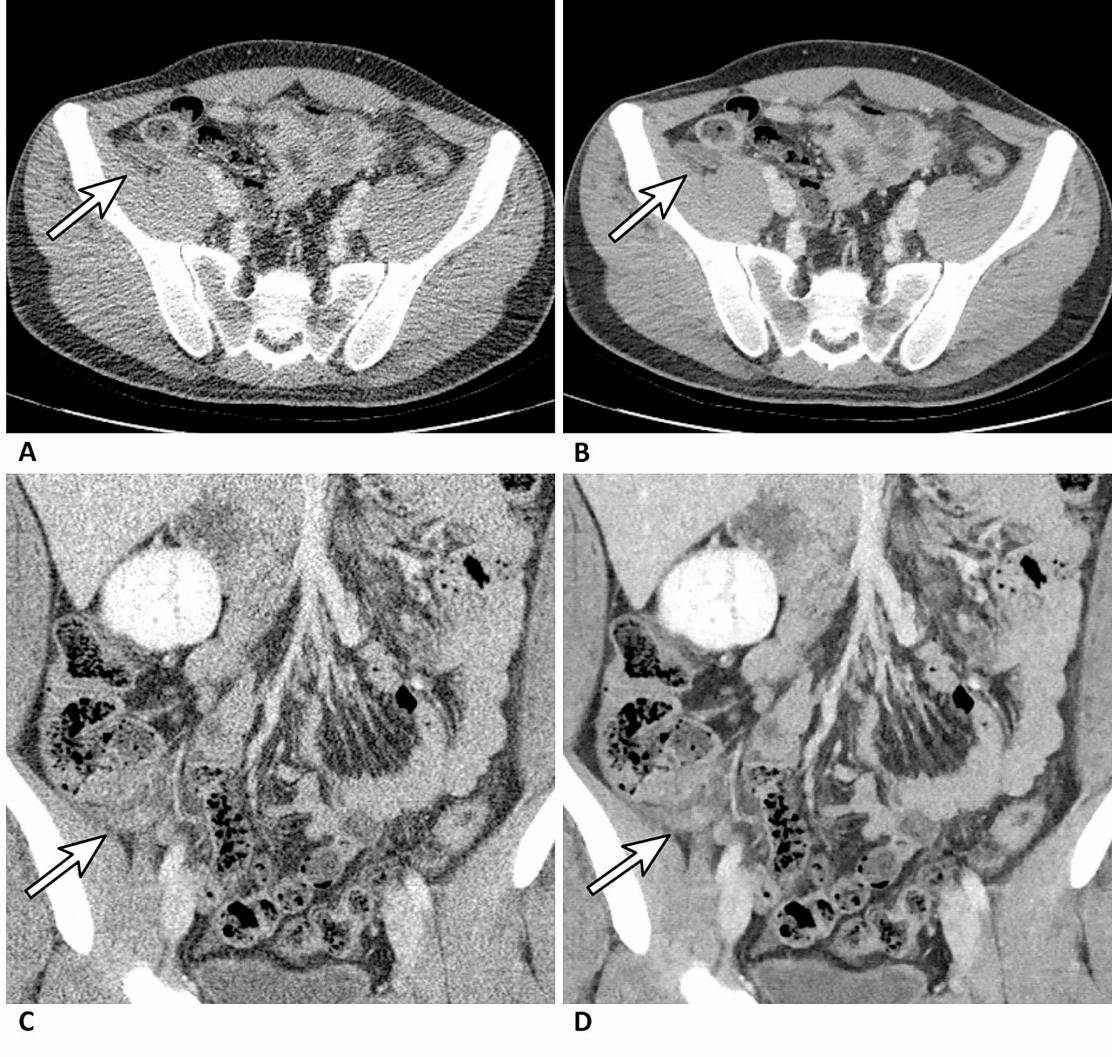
Representative images that an image-based deep-learning denoising algorithm improved diagnostic accuracy. A 37-year-old woman underwent a CT examination for suspected appendicitis (64-channel CT from Siemens). A second-year radiology resident (reader 6) diagnosed terminal ileitis on ultralow-dose CT images (a, c) and appendicitis on ultralow-dose CT with deep-learning denoising algorithm (b, d). The patient was confirmed with appendicitis through an appendectomy
